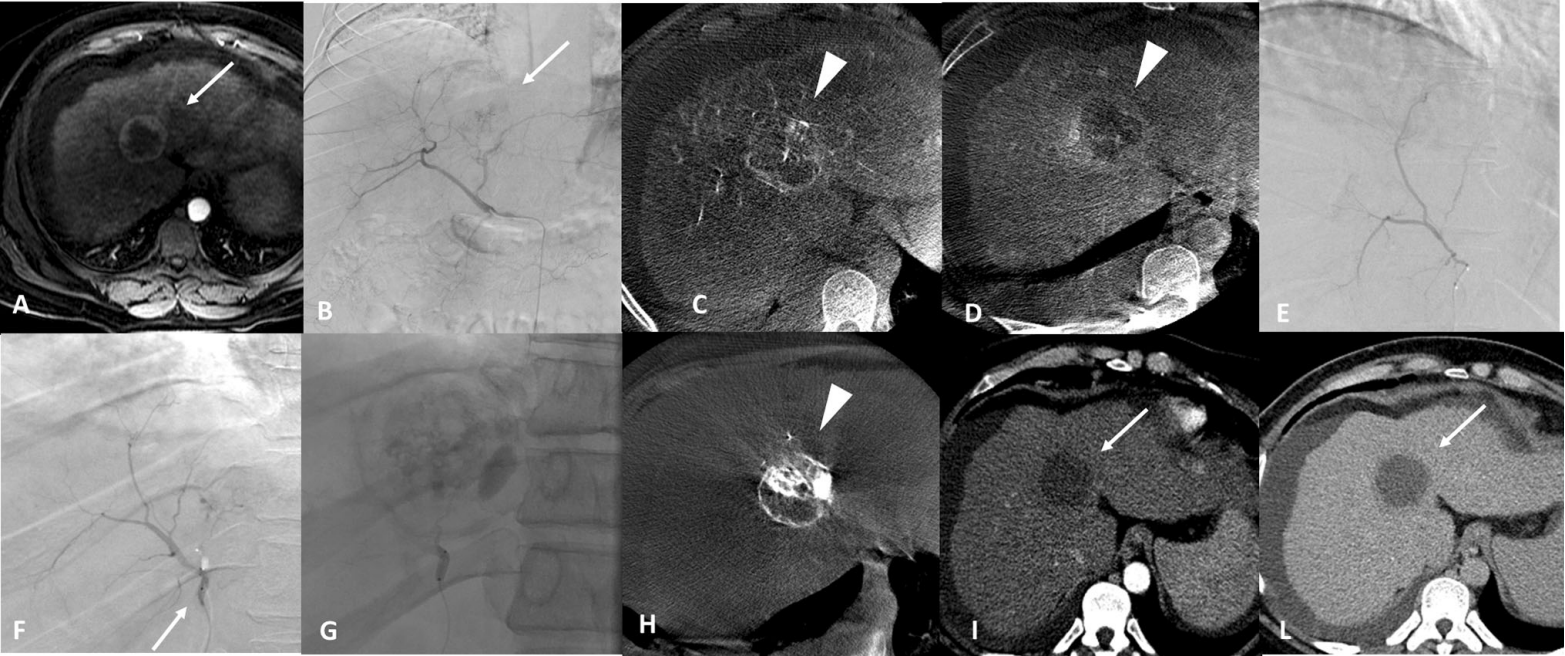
A 42-year-old female with HCC at level of the IV hepatic segment (maximal diameter 42 mm). Figure A, magnetic resonance imaging, arterial phase, shows non-homogeneous hypervascular nodule in the IV segment (arrow). The tumour is confirmed by the digital subtracted angiography (DSA) (figure B, arrow), in the cone-beam CT arterial phase (figure C) (arrowhead) and cone-beam CT delayed phase (figure D) (arrowhead). Super-selective DSA of the tumour with deflated balloon figure E) and inflated balloon (arrow) figure F). Figure G shows single fluoroscopy image after the embolization. Figure H non-enhanced cone-beam CT at the end of the procedure shows complete filling of the HCC (qTCR) (arrowhead). 1-month follow-up CT in arterial phase (figure I) and late phase (figure L) demonstrates the complete response of the HCC (arrow)
